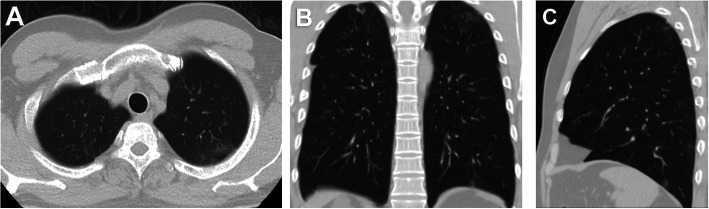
A 53-year-old female patient. A axial, B coronal, and C sagittal chest CT images show bilateral peripheral small ground-glass opacities with minimal pulmonary involvement (< 5%) corresponds to current chest CT score 4 and a simple chest CT score 1 (minimal affection). The patient survived
